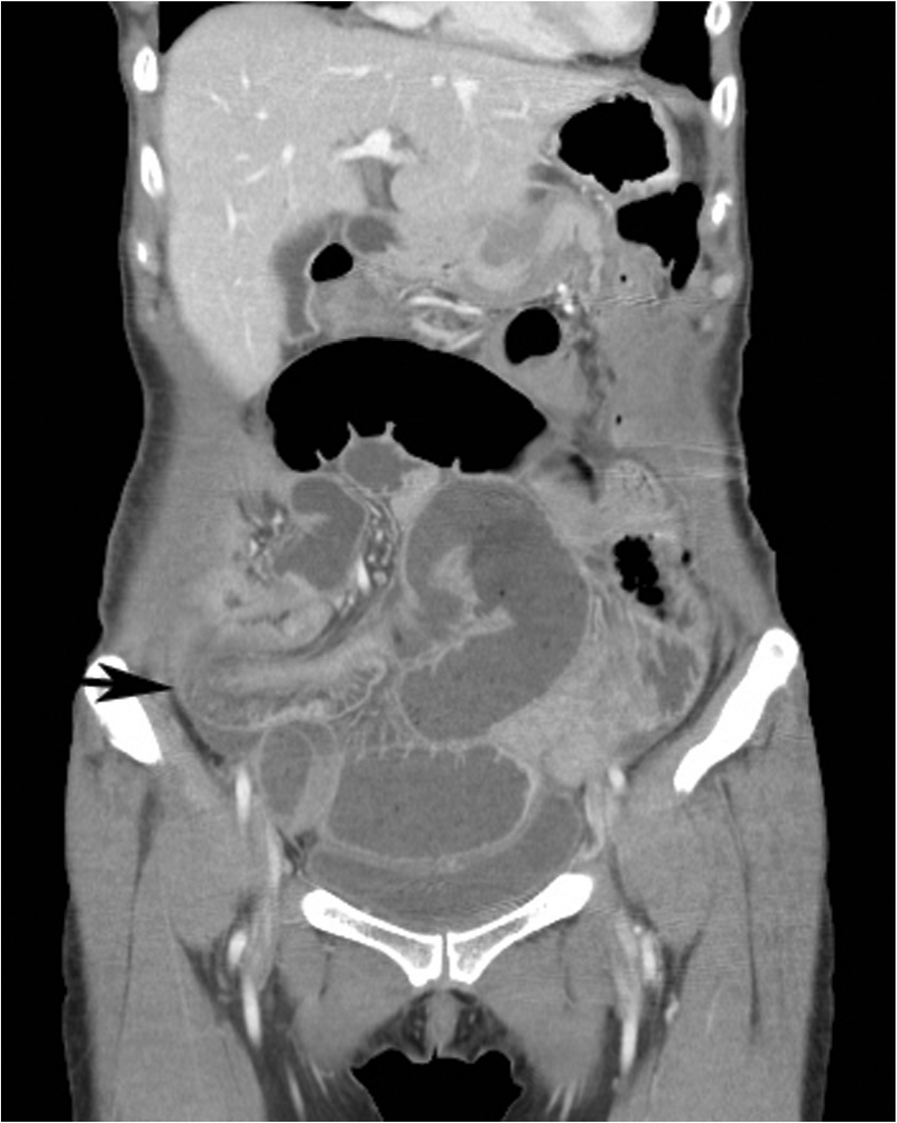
A follow-up abdominal computed tomography (CT) scan after laparoscopic low anterior resection for rectal cancer on postoperative day 3. A coronal image showing ileo-ileal type intussusception (*black arrow*)
